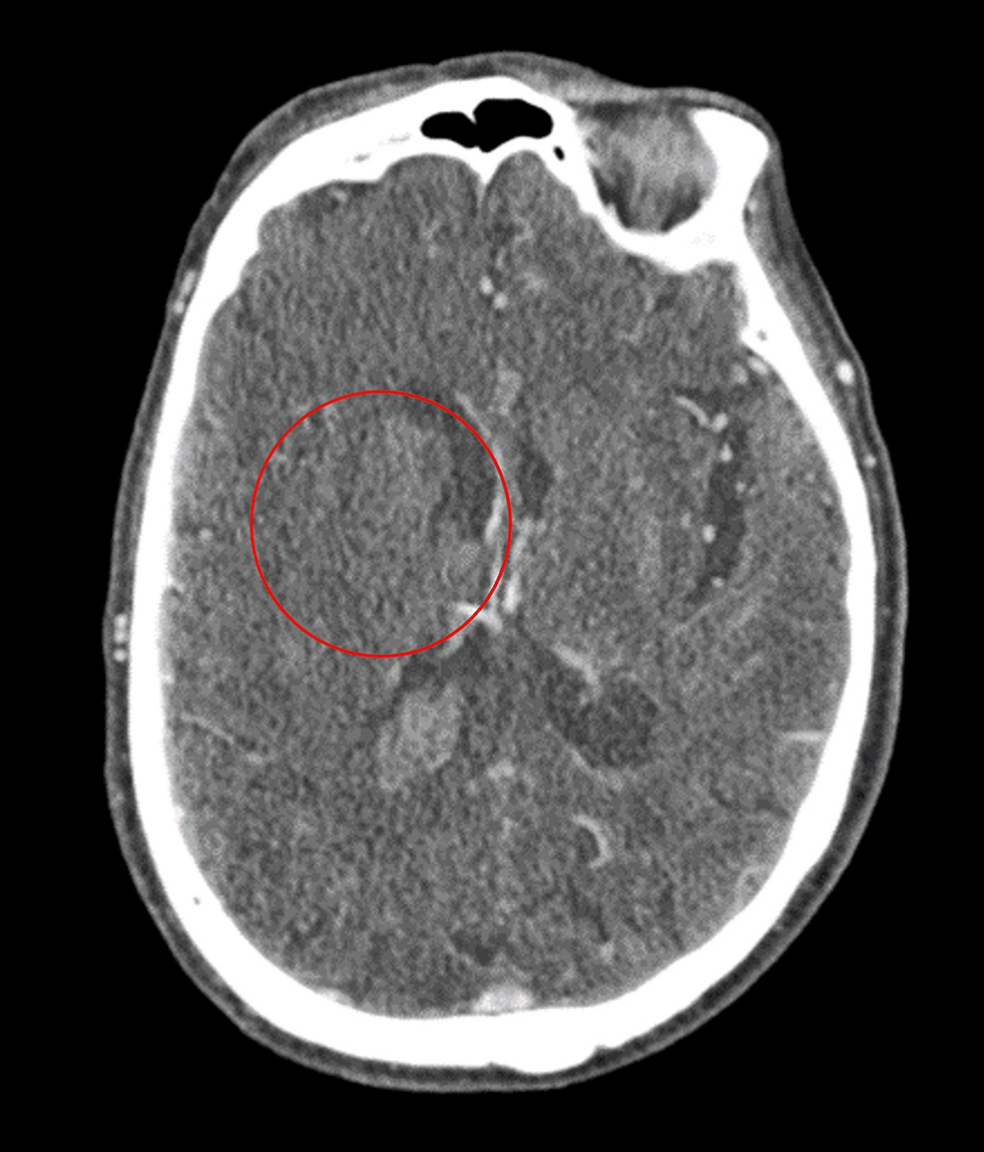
Initial CT head without contrast in the axial view showing acute hemorrhage in the paramedian right frontal lobe (red circle) with extension into the right lateral ventricle.
